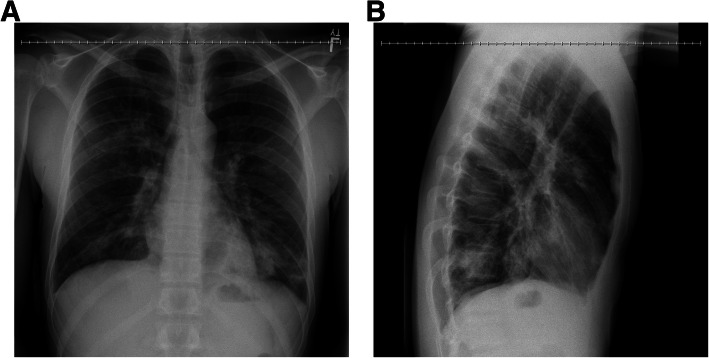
Chest x-ray on admission; PA and lateral view. Patchy infiltrates can be seen in both lower lobes and the right upper lobe
